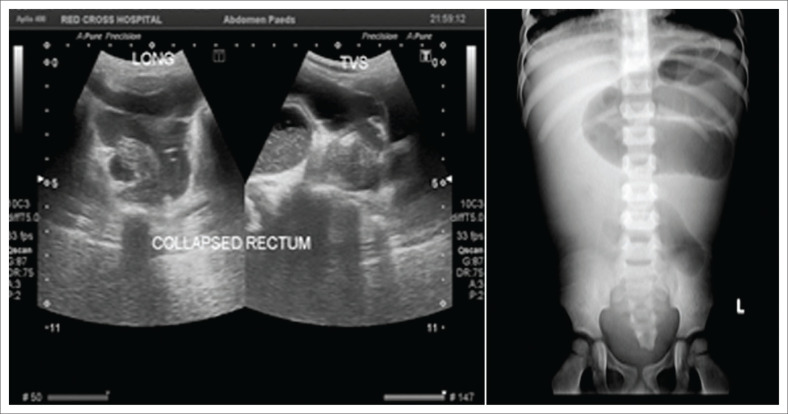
Ultrasound and supine abdominal X-ray showing markedly distended small bowel loops and collapsed rectum.
